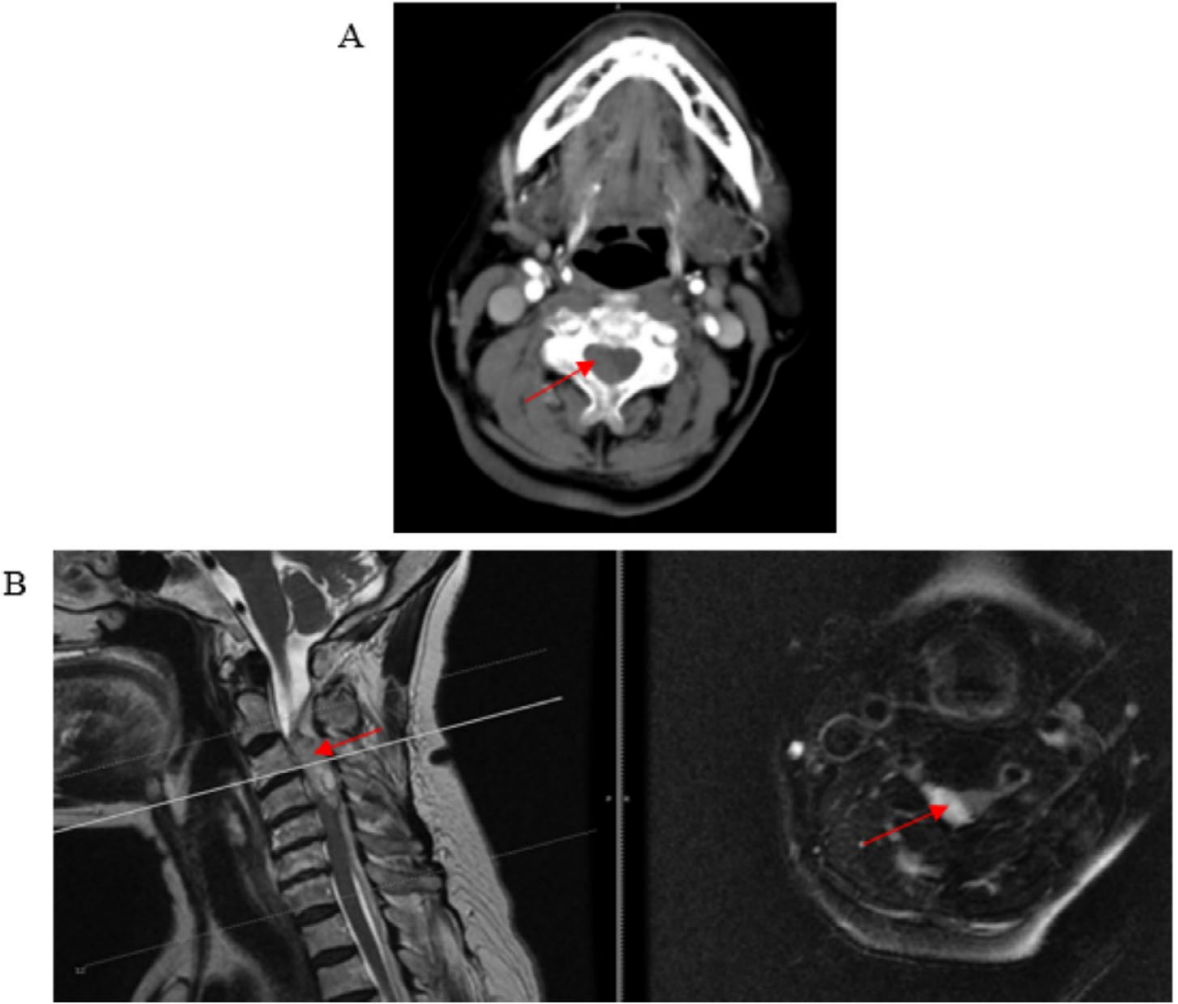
(A) Cervical CT scan reveals an epidural hematoma with significant compression and flattening of the spinal cord. (B) Cervical MRI demonstrates an epidural hematoma within the cervical spinal canal, showing leftward displacement of the spinal cord at the corresponding levels, most notably at C2–5.
